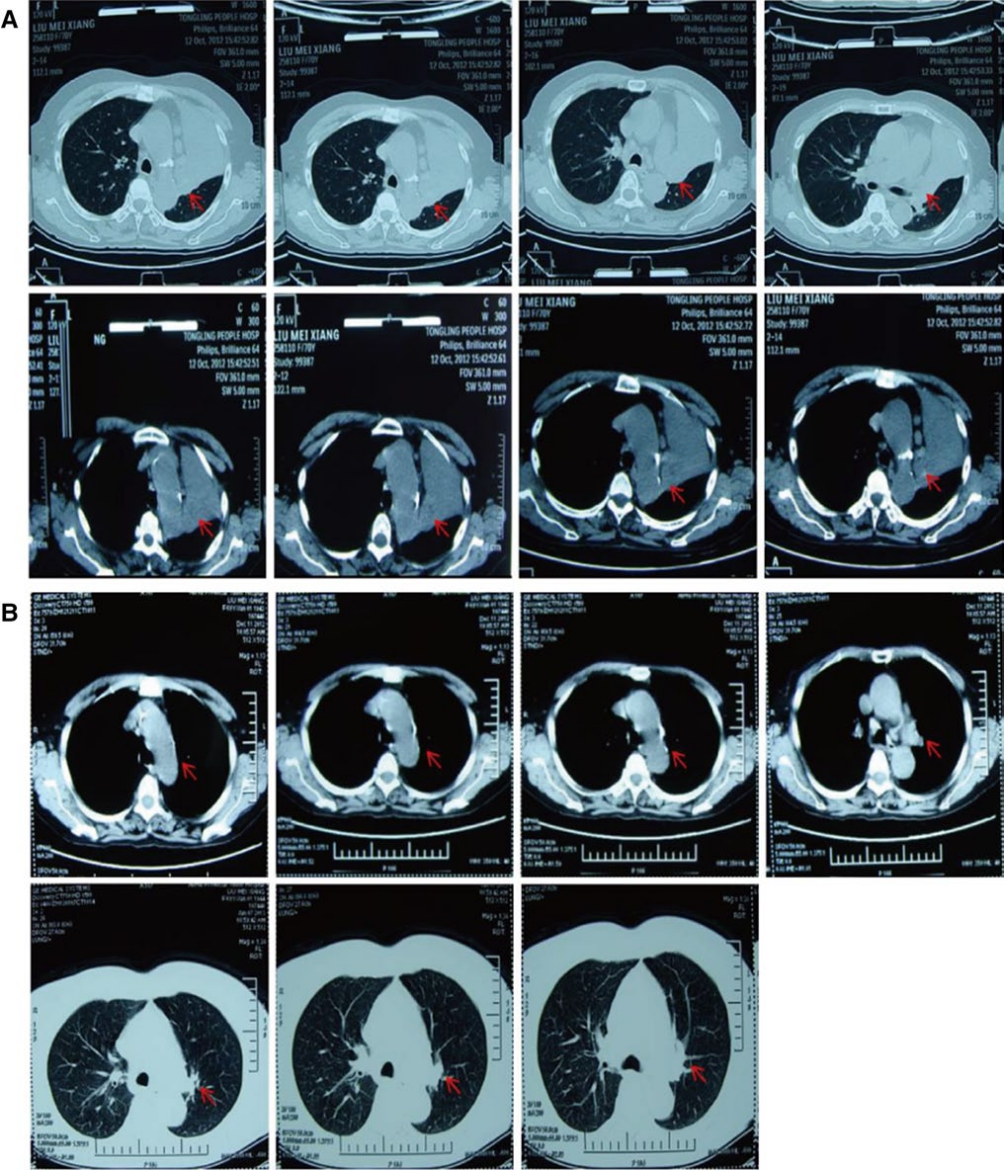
CT scan of the patient with moderately differentiated adenocarcinoma of the *left* lung complicated by atelectasis before (a) and after (b) interventional therapy. The *arrows* showed the position of lesions
